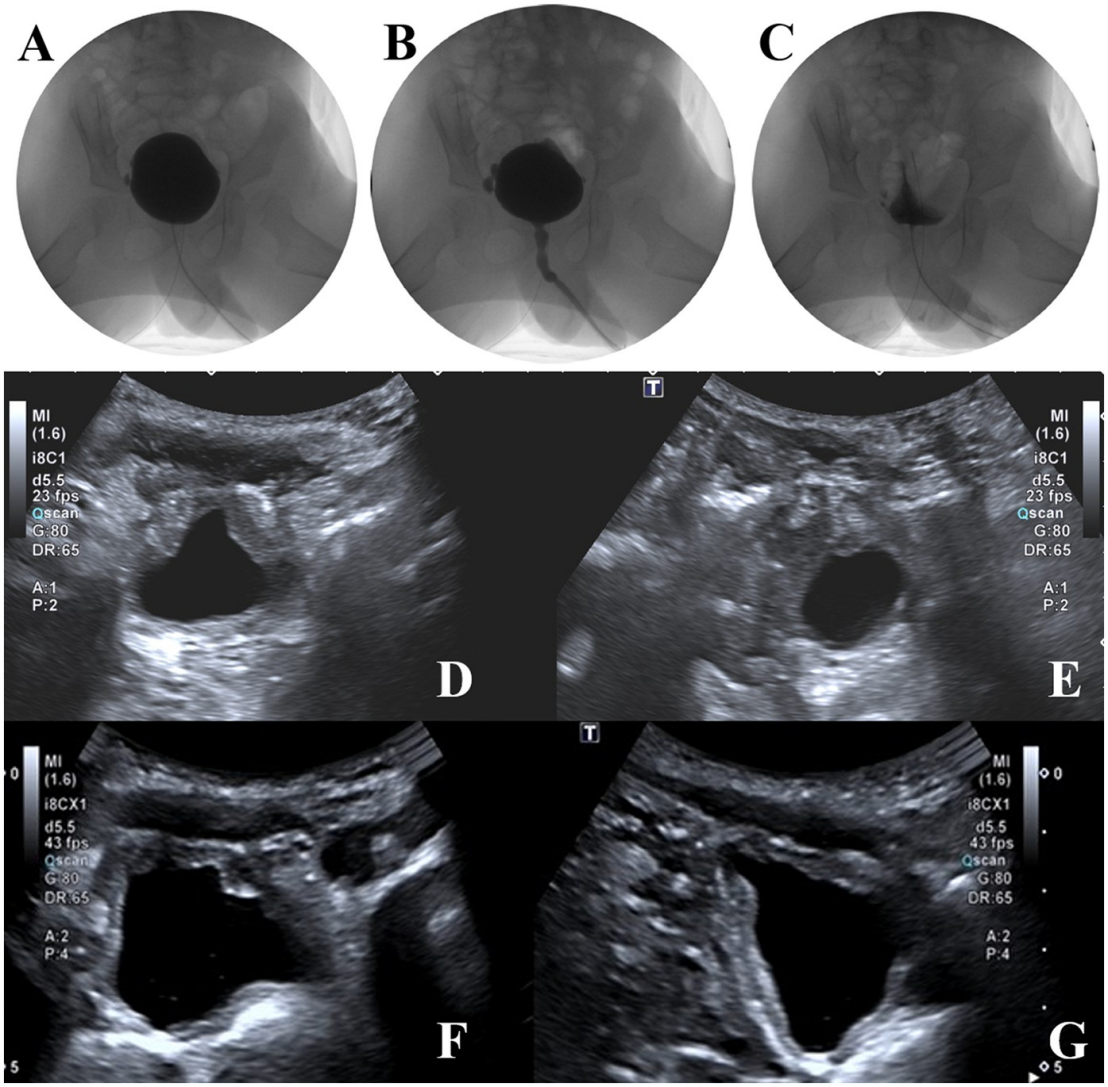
Postoperative video urodynamic study images showing the bladder filling phase (A), voiding phase (B), and post-void phase (C) follow-up ultrasound images demonstrating no new diverticula and improved bladder morphology (D–G).
